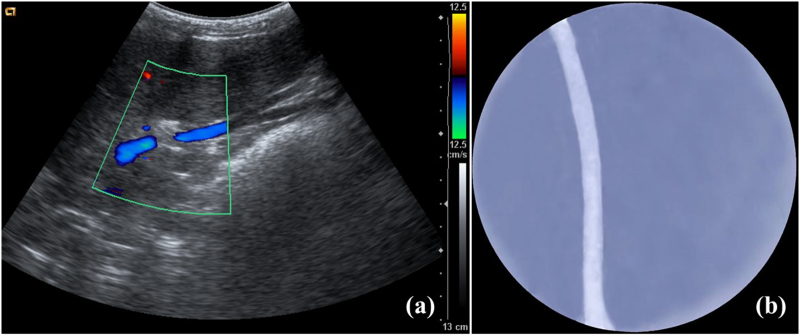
Representative imaging findings after surgery in the M-OLT group. (a) PV ultrasound image; (b) IVC angiographic image.
